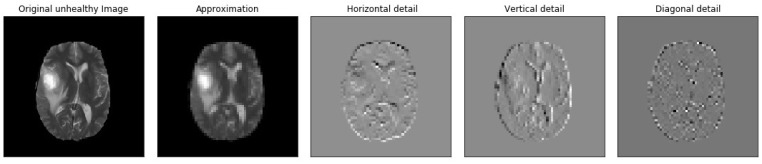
Samples of our Level 2 DWT decomposition of a non-healthy MR image type T2.
